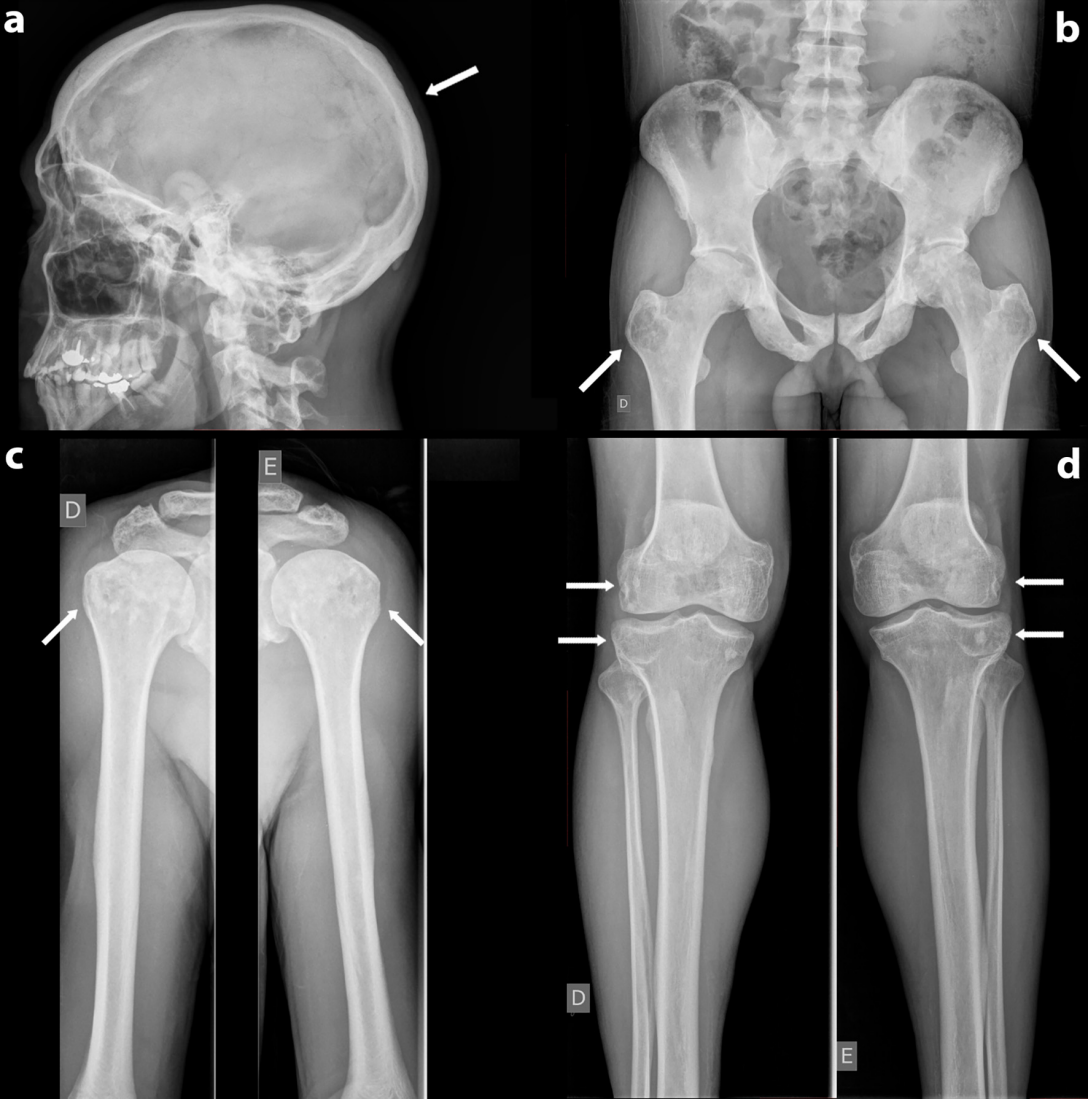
(A) Normal skull radiography. (B) Anteroposterior radiography of the pelvis Symmetric radiolucency with cystic aspect in greater trochanter bilaterally. (C) Anteroposterior radiography of upper limbs- heterogeneous bone density in humeral heads bilaterally with the presence of sclerosis and radiolucent areas. (D) Anteroposterior radiography of lower limbs-pseudo-poikilosis (patchy sclerotic lesions) and radiolucency in distal metaphysis of right and left femur and in proximal region of tibia bilaterally.
